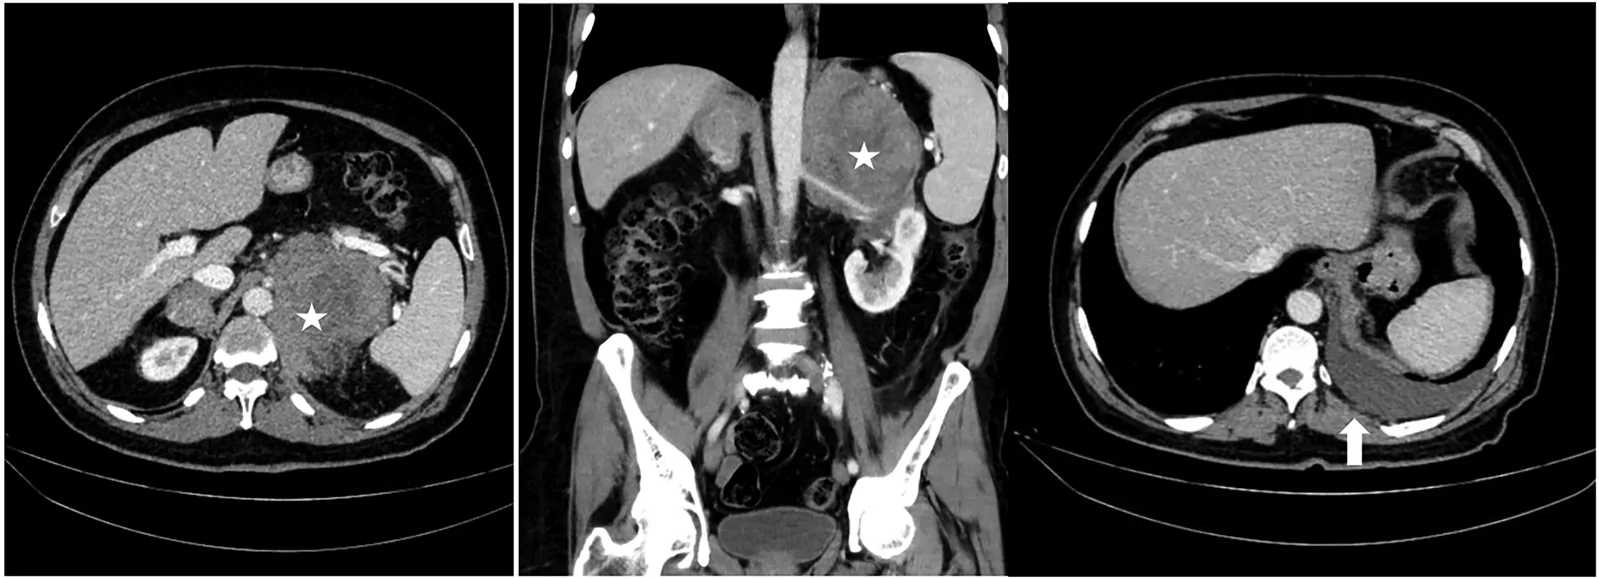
Contrast-enhanced abdominal CT axial and coronal images showing bilateral adrenal masses(asterisk) with hemorrhagic components at different stages. Axial contrast-enhanced chest CT scan showing left-sided pleural effusion (arrow) associated with chylothorax, confirmed by pleural fluid biochemical analysis.
